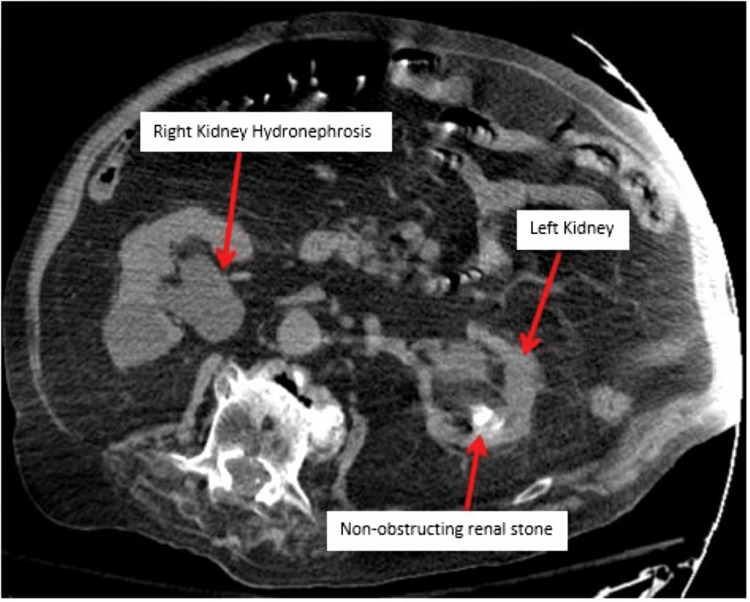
Abdominal CT demonstrating right kidney hydronephrosis, and non-obstructive renal stone in left kidney
